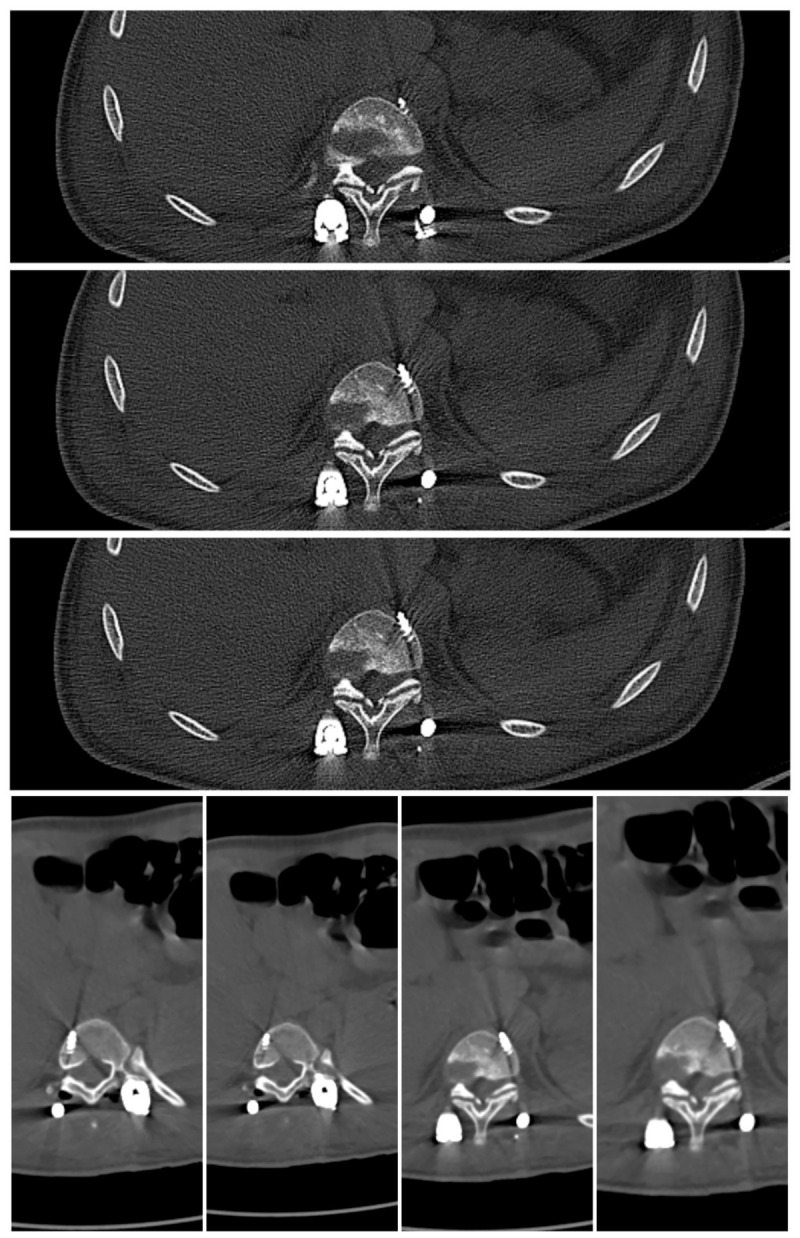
CT at the T10 level in a patient with achondroplasia showing the characteristic congenitally narrow spinal canal and dysplastic, narrowed pedicles. A left-sided transpedicular screw traverses the pedicle with anterior and lateral cortical breach of the vertebral body; the screw tip projects ~5 mm beyond the cortex, in close proximity to the thoracic aorta. The screw was removed during revision surgery.
